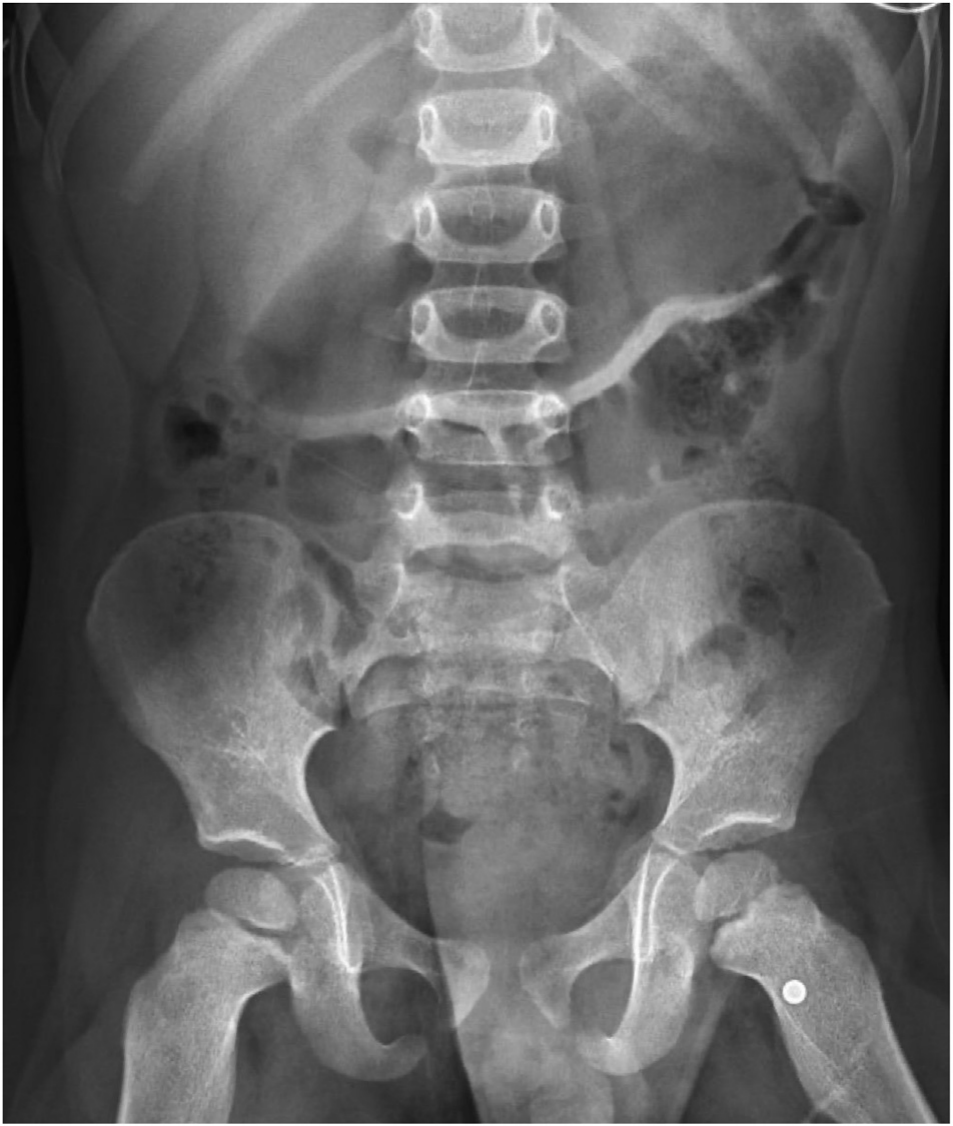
Plain radiograph of the abdomen showing the pellet overlying the left groin.
